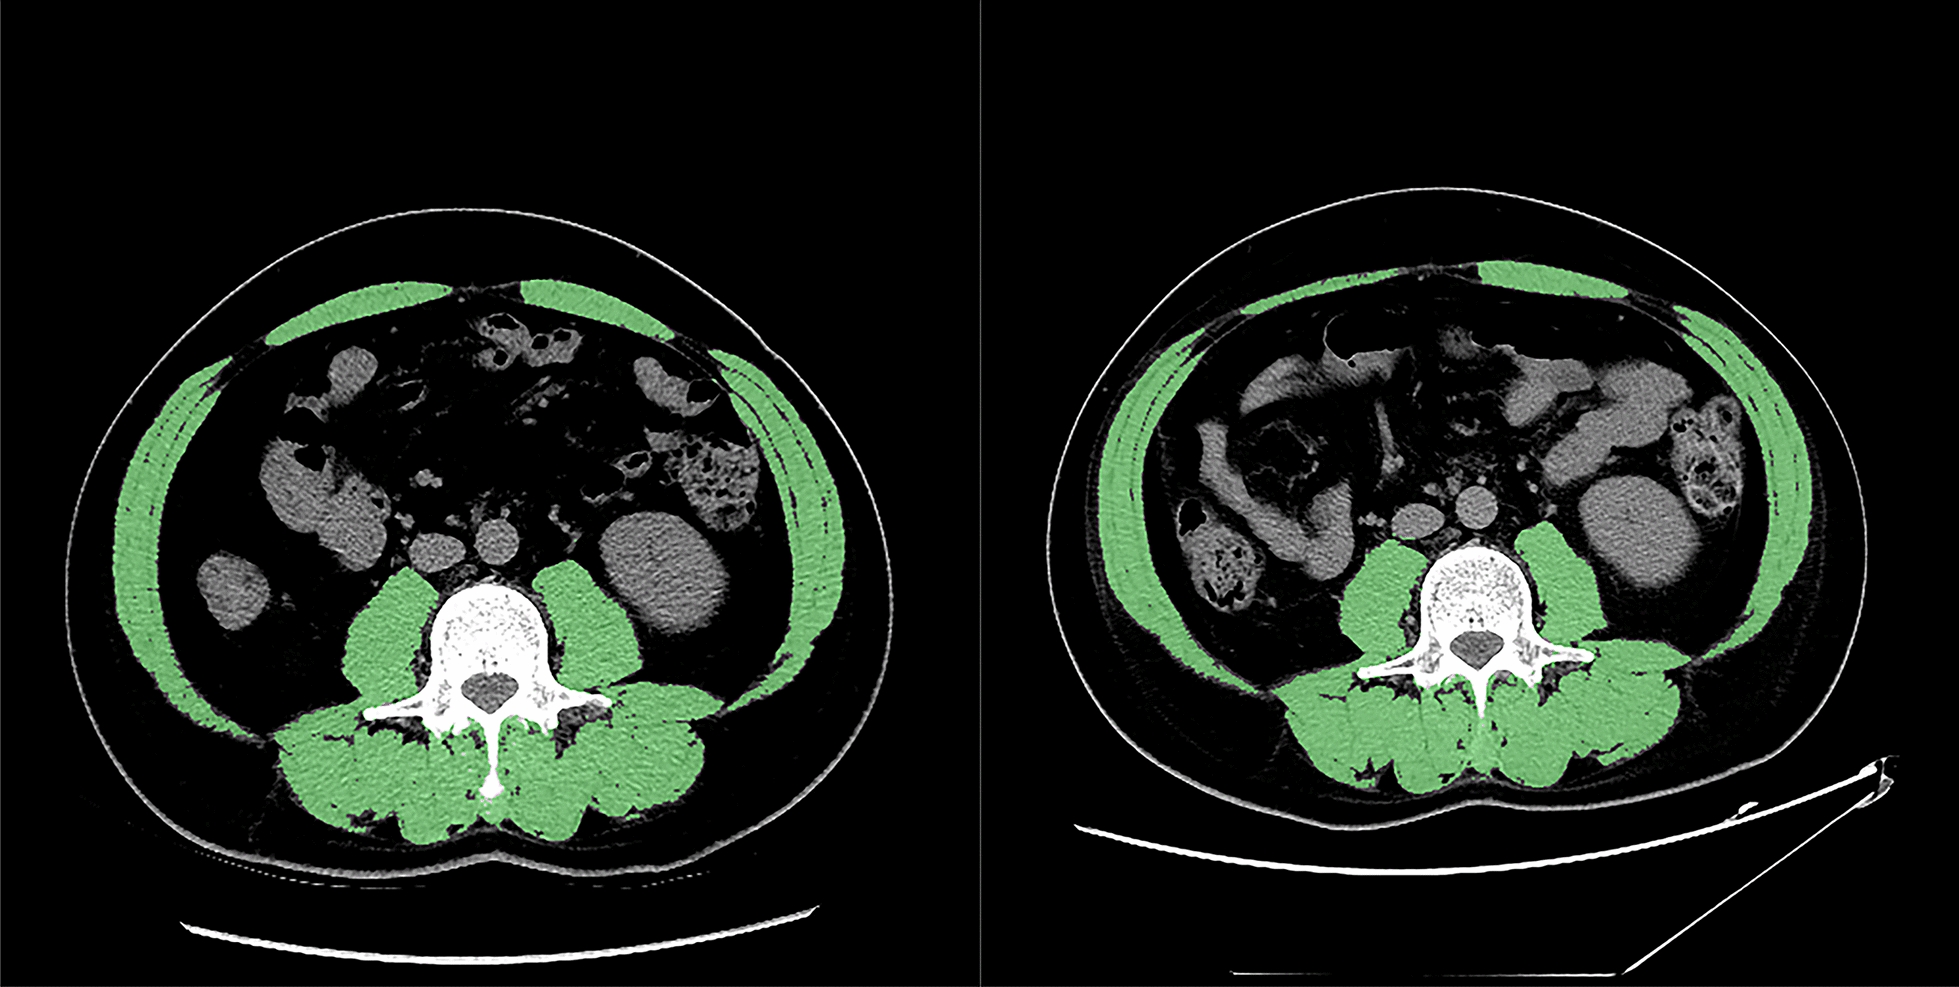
Skeletal muscle loss marked by green mask over 171 days in a 52-year-old man with a single moderate differentiated HCC lesion. a Preoperative skeletal muscle index (SMI) on axial CT image at the L3 level was 46.97 cm^2^/m^2^. b Postoperative SMI at the same plane on CT image was 40.82 cm^2^/m^2^
